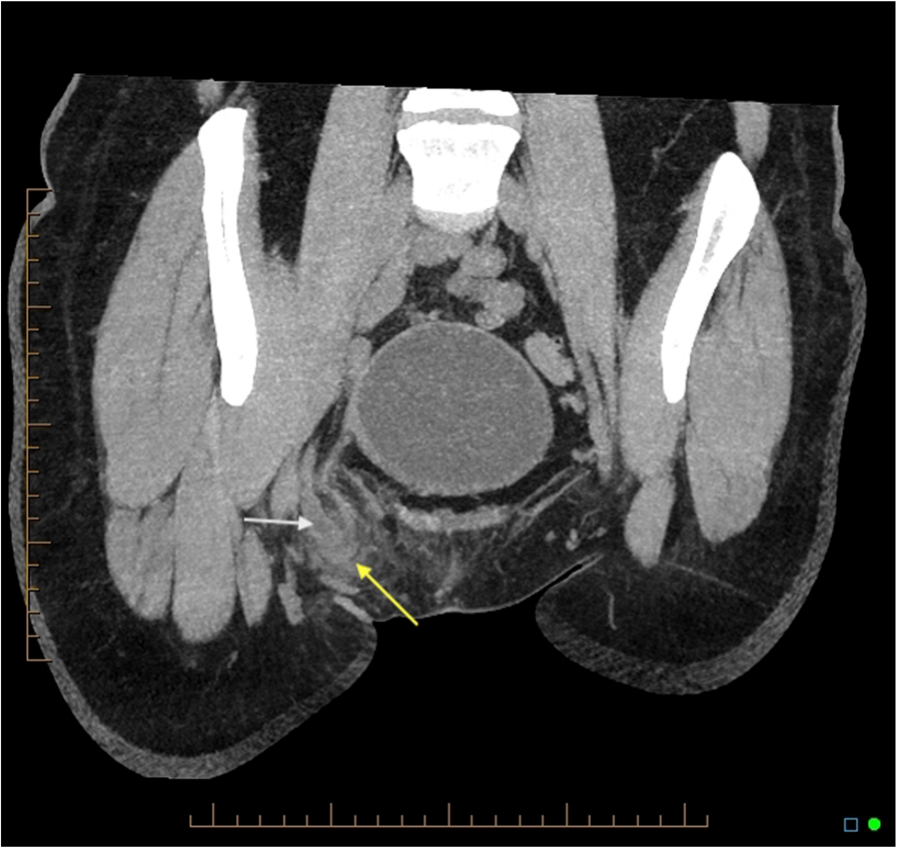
CT scan coronal view shows appendicitis (white arrow) inside the femoral canal (yellow arrow)
